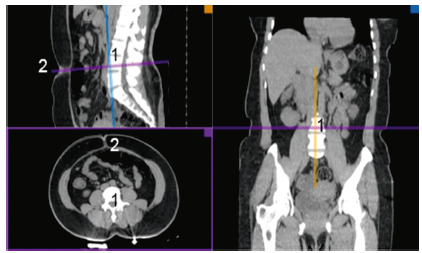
Standardization of the cut level on the CT to quantify the fat.
